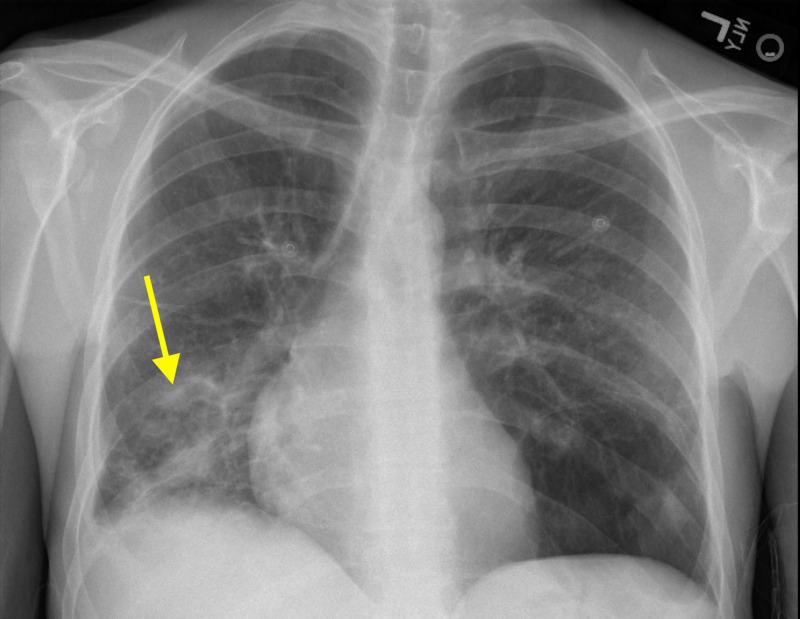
Chest X-ray depicting a predominantly right lower lung infiltrate (yellow arrow) concerning for a developing infectious process.
